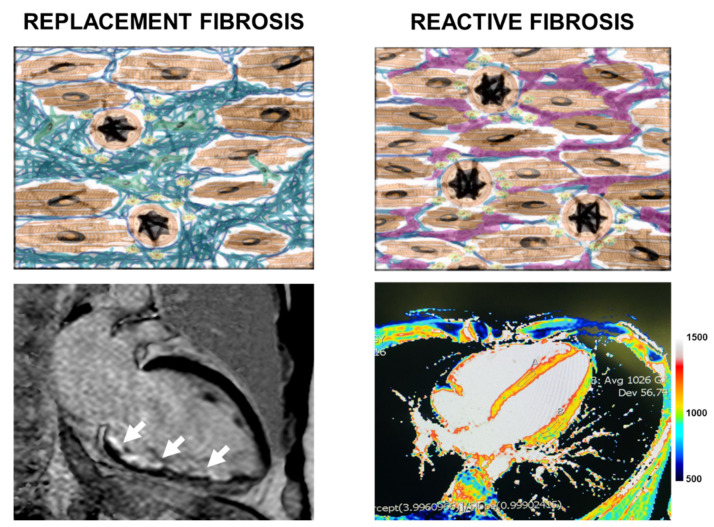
Description of the main patterns of myocardial fibrosis and the corresponding cardiac magnetic resonance images. Right panel: replacement fibrosis occurs in response to cardiomyocyte loss and can be observed in LGE image as a regional subendocardial scar (white arrows). Left panel: reactive fibrosis occurs without cardiomyocyte loss and can be observed in native T1 mapping image as raised native T1 values (1000–1200 ms).
